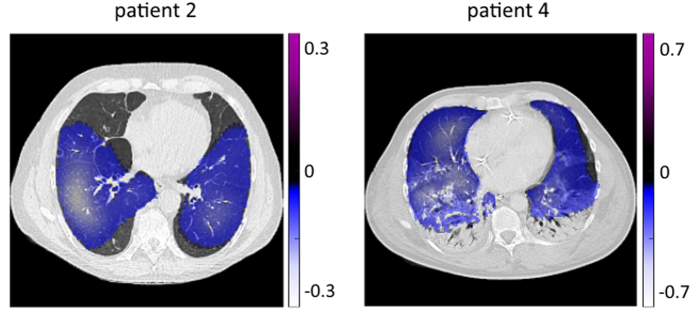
Superposition of changes in conductivity distribution obtained by the described DCT approach and CT images in a patient with emphysema in the ventral lungs (left) and atelectasis in dorsal lungs (right).
